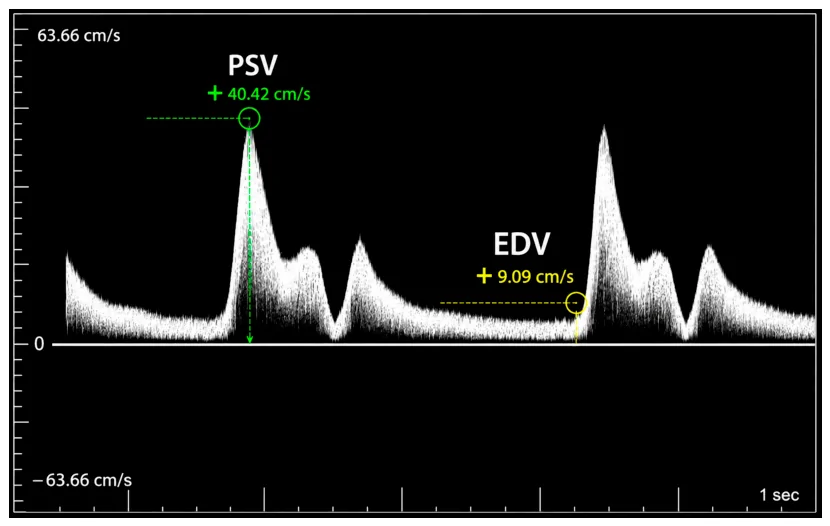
Analyzed Pulsed-Wave Doppler Ultrasound Image of Temporal Artery.
